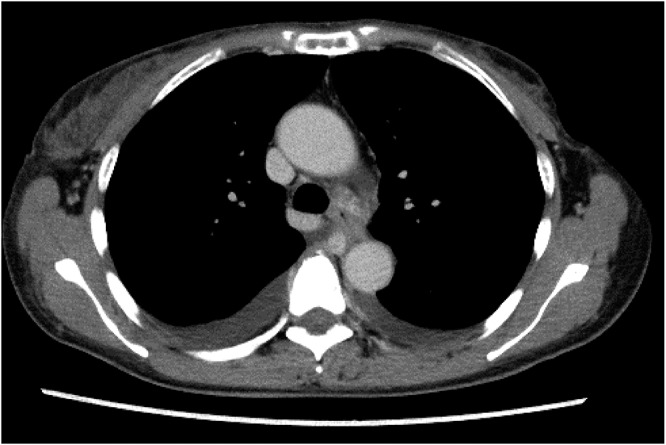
Chest computed tomography scan showing continued reduction in bilateral pleural effusions.
